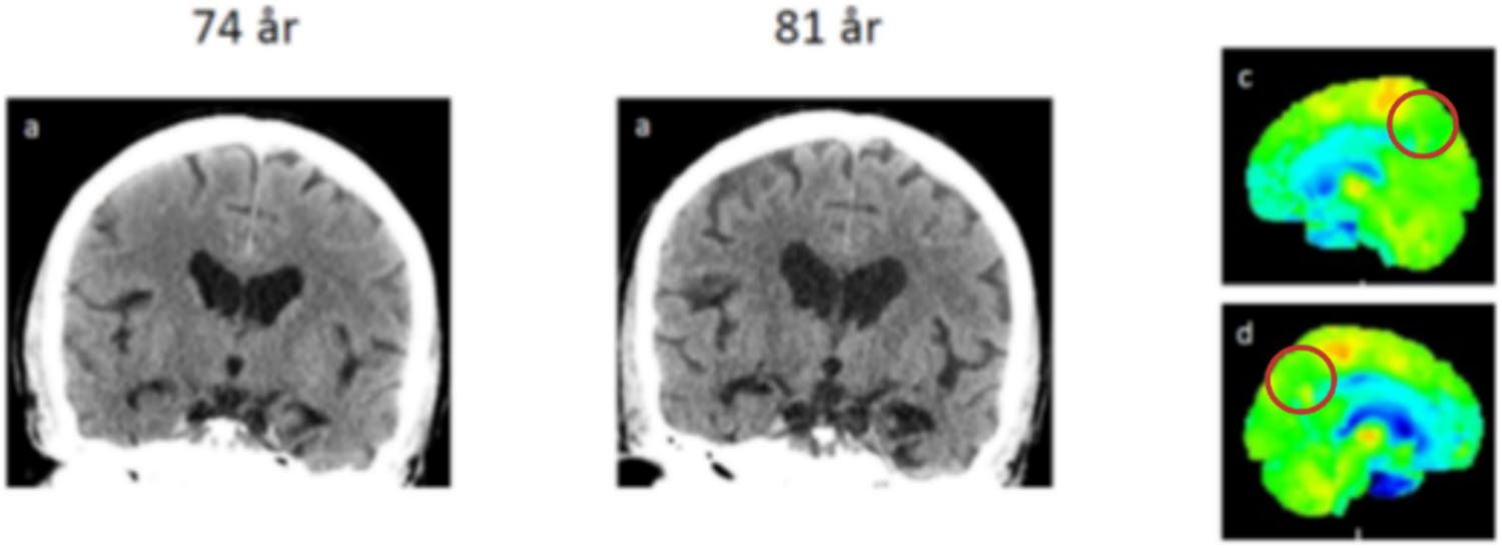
Baseline (left) and 7 years follow up CT of the brain (right) indicating accelerated atrophy of anterior hippocampus but not the cortex. [^18^F]FDG-PET showed accelerated hypometabolism (blue color) in the temporal lobe and medial frontal/cingulate cortex but not in the typical AD regions as precuneus (red ring)
